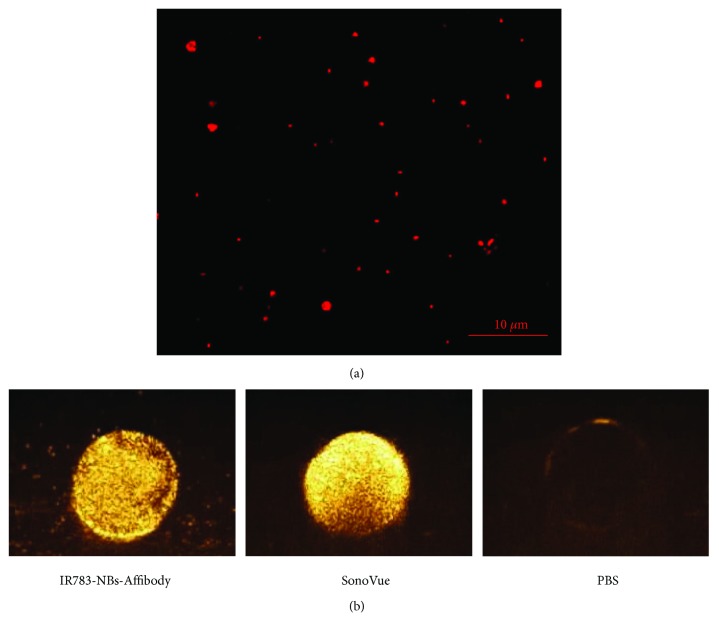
NIRF and ultrasound bimodal images of IR783-NBs-Affibody *in vitro*. (a) A LSCM image of IR783-NBs-Affibody. (b) The contrast-enhanced ultrasound images of IR783-NBs-Affibody, SonoVue microbubbles, and PBS *in vitro*, respectively.
